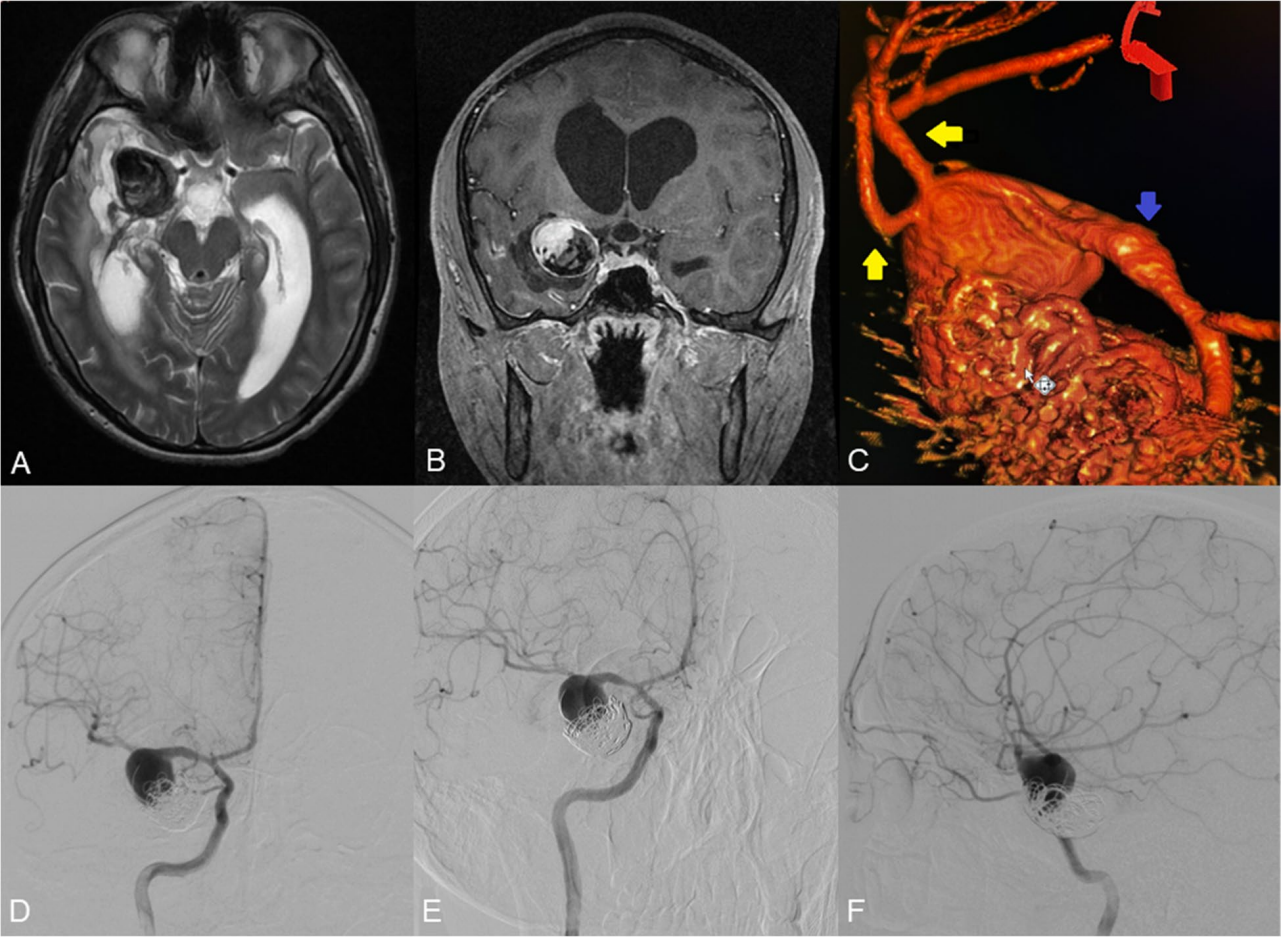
Axial (A) T2-weighted and frontal (B) T1 contrast-enhanced MRI showing the giant partially thrombosed intracranial aneurysm of the left MCA. C 3 d reconstruction of the aneurysm (The blue arrow indicates the M1 segment of MCA, and the yellow arrows indicate the M2 branches of MCA originating from the aneurysm dome). A DSA from the left ICA (D-F) shows a giant partially coiled aneurysm of the left MCA
